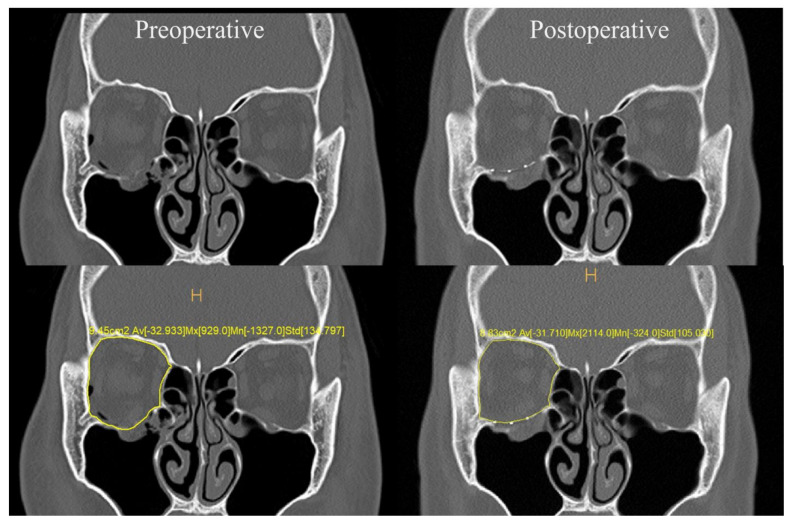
Preoperative and postoperative CT. Soft tissue herniation is observed in right inferior orbital wall fracture. Synpor^®^ porous polyethylene implant containing titanium was used because of the wide fracture area. The implant was fixed using a tongue-in-groove technique. Postoperative CT shows a well-positioned implant compared to the unaffected side. Deja-view software was used to calculate the volume of both orbits (yellow).
